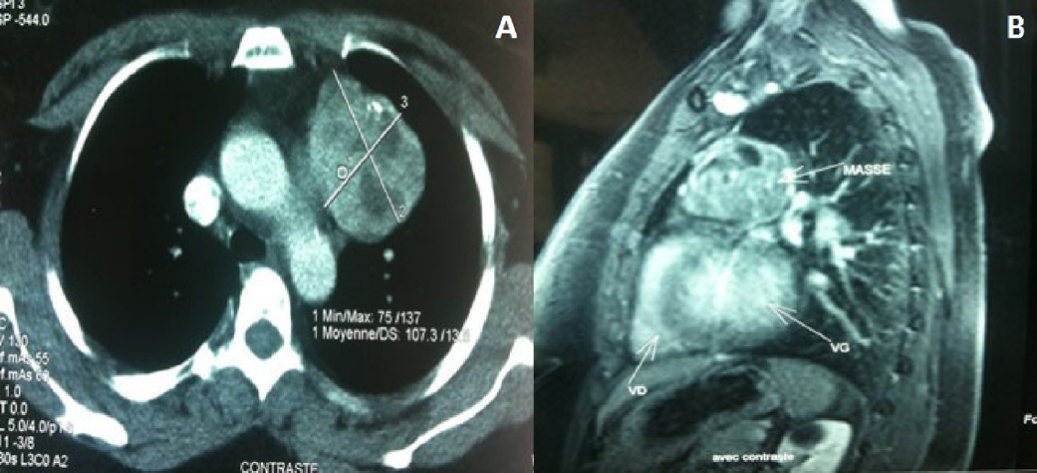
Inhomogeneous multicystic mass lesion in the left mediastinum, tightly adherent to the aortic arch, pulmonary artery and left ventricle on CT scan (A) and MRI (B).
